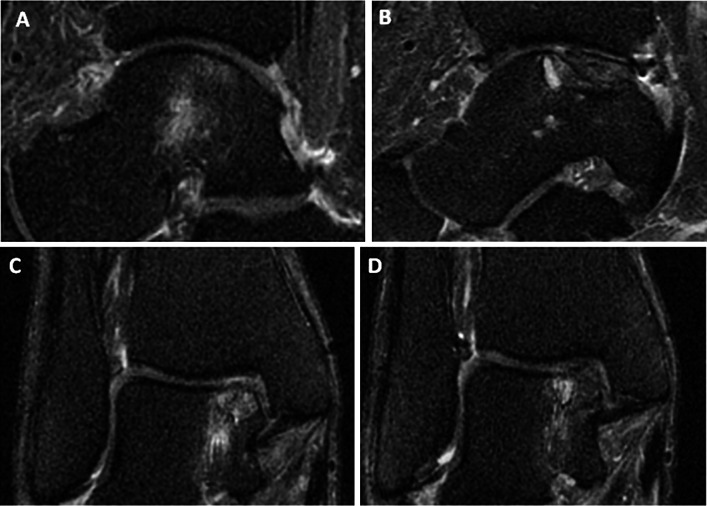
Magnetic resonance imaging (MRI) scans. a
